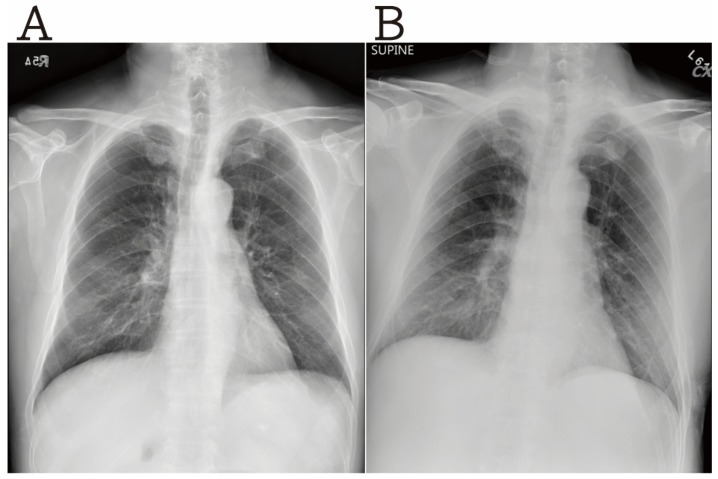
The chest X-ray revealed mild increased bilateral lung markings without a significant pneumonia patch (A) initially (B) after one day.
